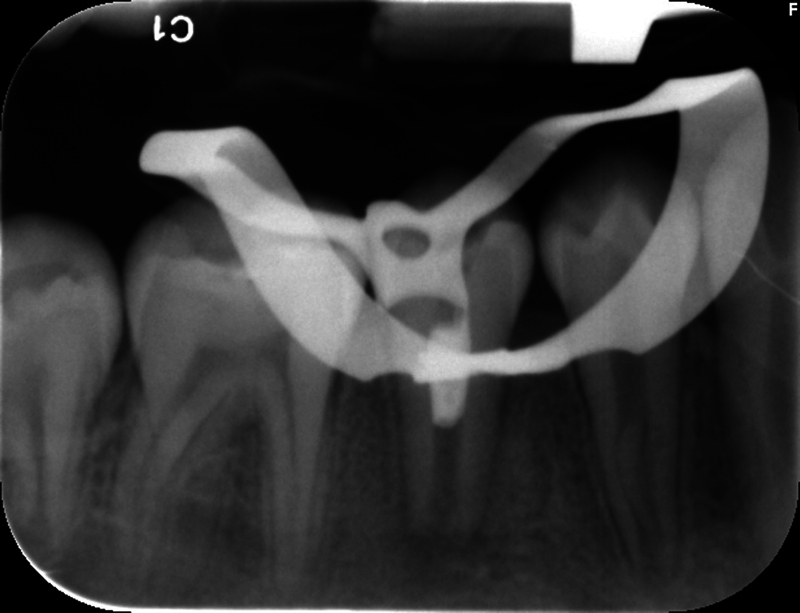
Obturation periapical radiograph with EndoSequence BC RRM Fast Set Putty.
